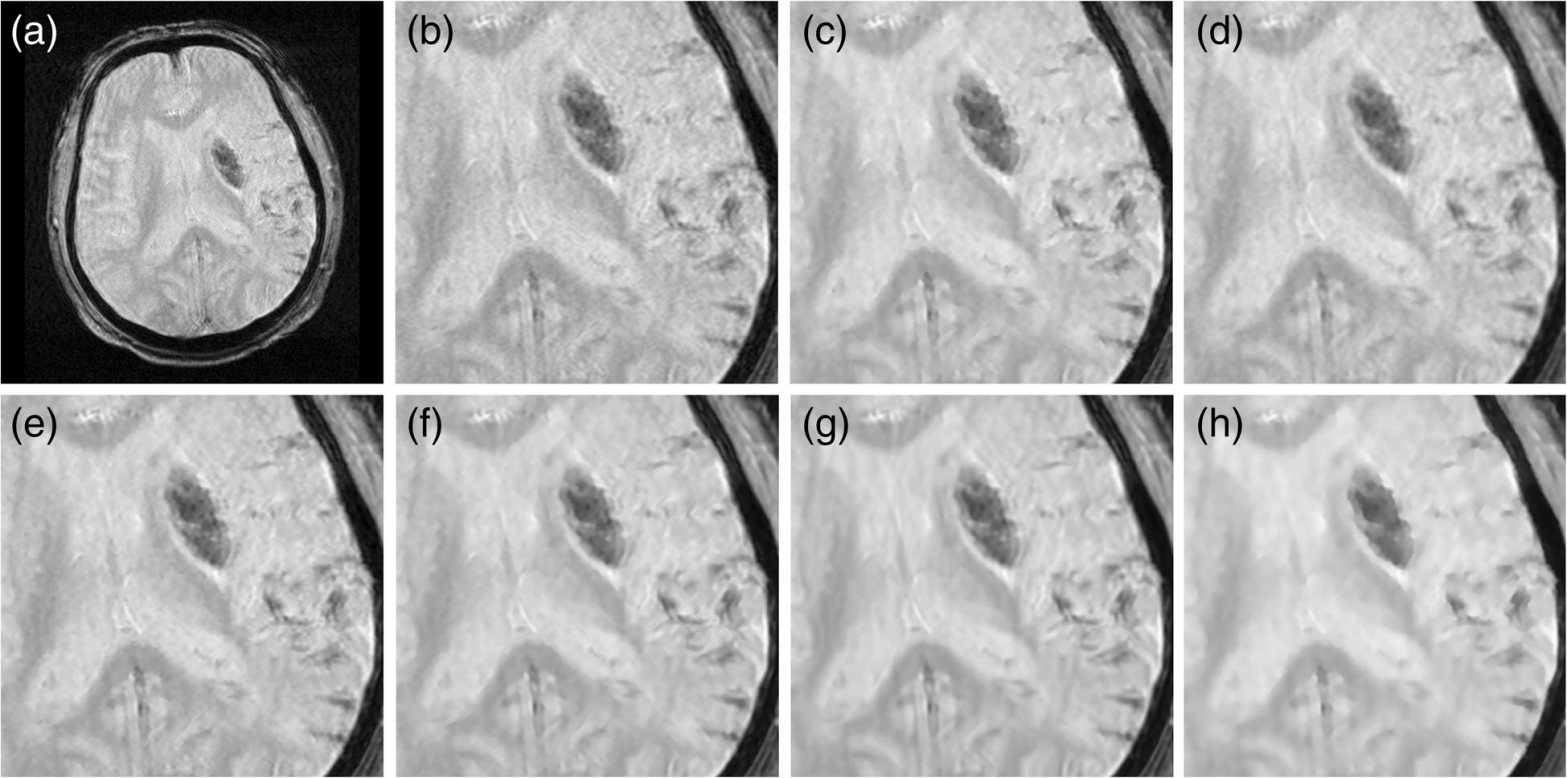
Comparison of the restoration of distorted PD-weighted MR images with brain tumors. a Input MR image. b Input image in magnified view. c IBF. d ADF. e LMMSE. f NLM. g BM3D. h Proposed
